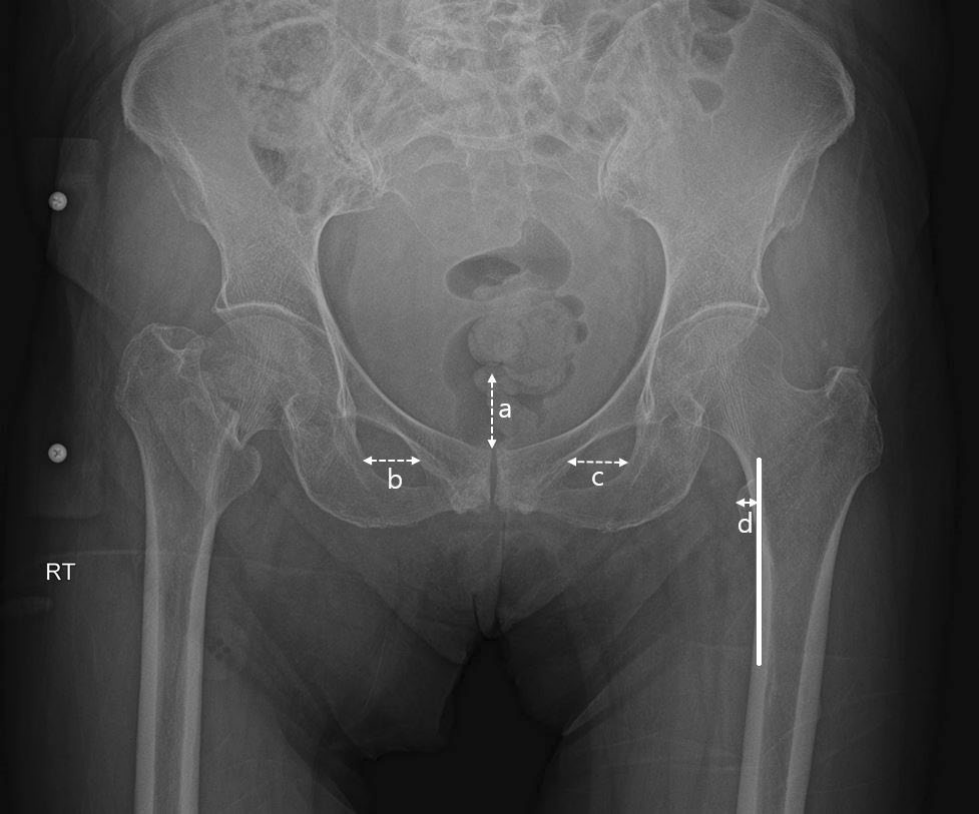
Quality assessment of a standard preoperative anteroposterior pelvic radiograph with femurs internally rotated by 15°. (a) Pelvic tilt was assessed by the distance from the pubis symphysis to the tip of the coccyx. (b) and (c) Pelvic rotation was assessed by first measuring the horizontal diameters of both obturator foramina and then calculating the ratio of the two measurements. (d) Femur rotation was evaluated by measuring the lesser trochanter thickness.
